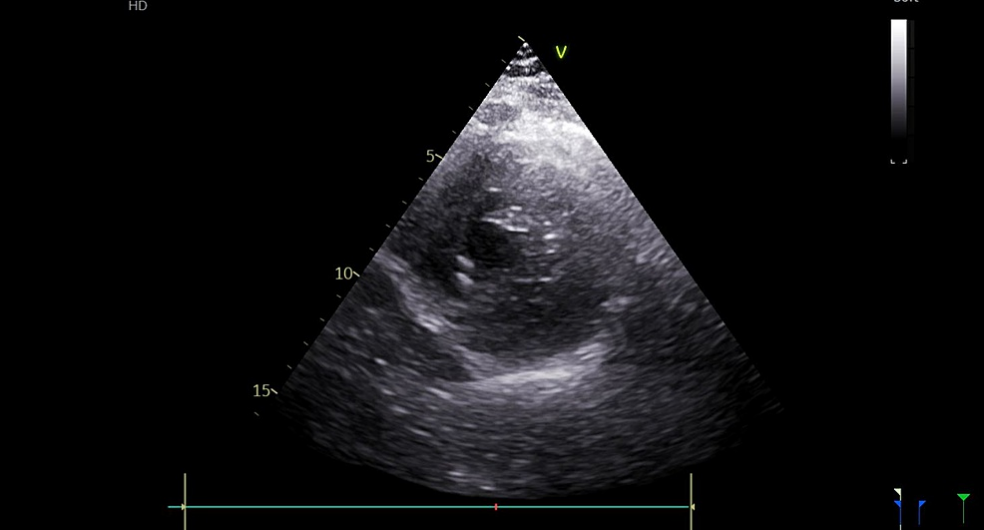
Echocardiogram in the systolic phase. Post-procedure transthoracic echocardiogram (TTE) showed a left ventricular ejection fraction (LVEF) of 44%, and hypokinesis of the mid to distal septum and apex.
